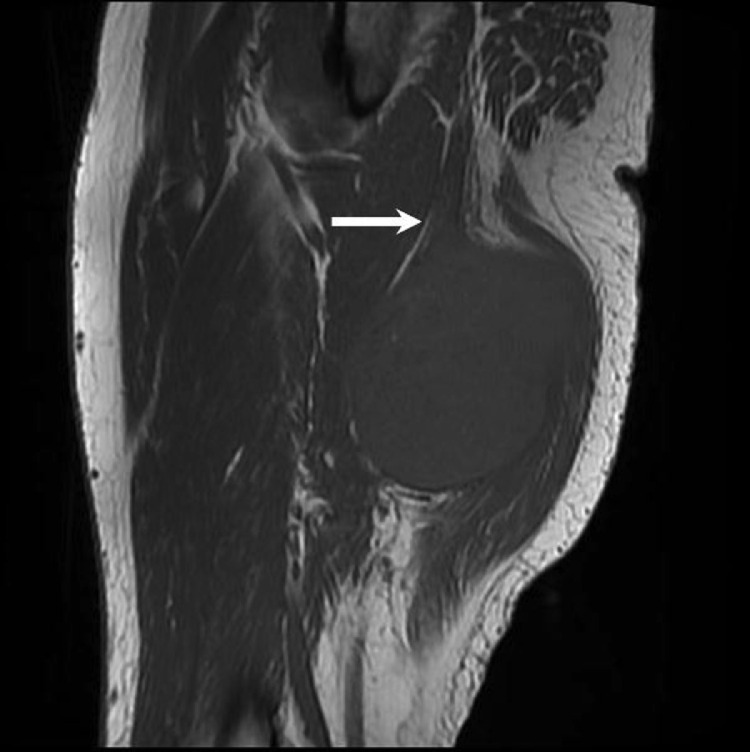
Sagittal T1 MRI image shows isointense T1 signal of the mass relative to skeletal muscle. There is no discernable fat plane between the mass and the sciatic nerve (arrow).
